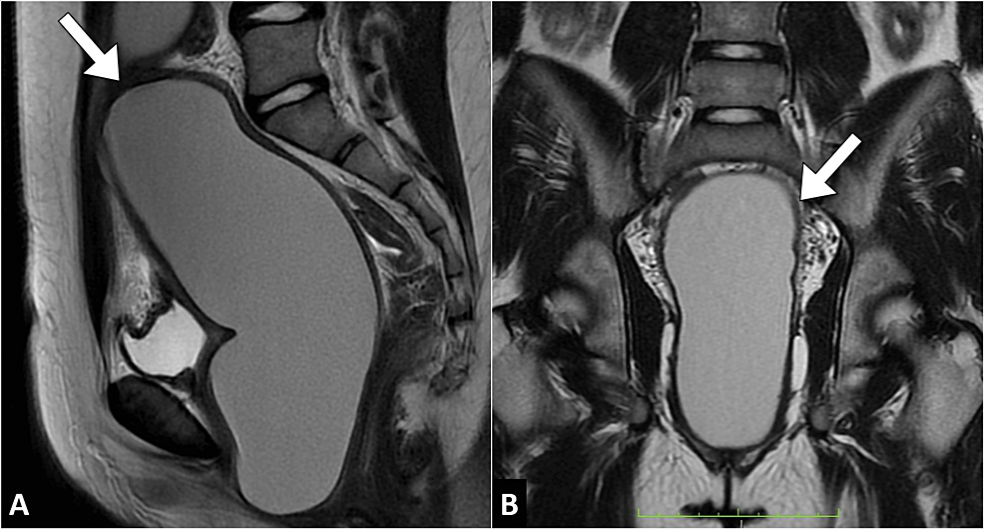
Sagittal (A) and coronal (B) T2-weighted MR images of the pelvis reveal an elongated and dilated uterus filled with blood products (arrow), consistent with hematometra. This condition exerts a notable mass effect in the pelvis, accompanied by the absence of the vagina. MR: magnetic resonance
